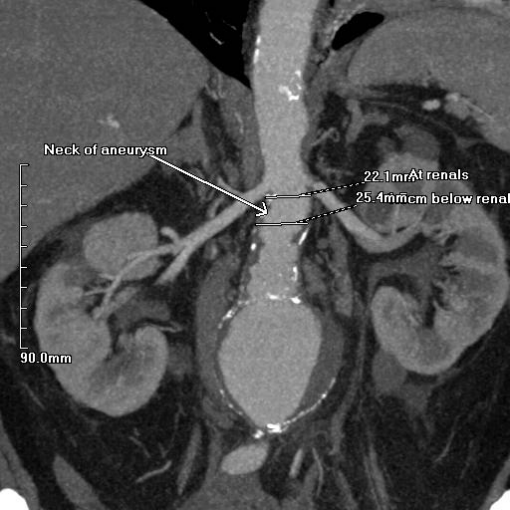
Coronal CT angiogram image demonstrating the 7.0 cm aneurysm. The neck of the aneurysm was not angulated and its diameter at the renal arteries was 22.1 mm and below the renal arteries was 25.4 mm.
